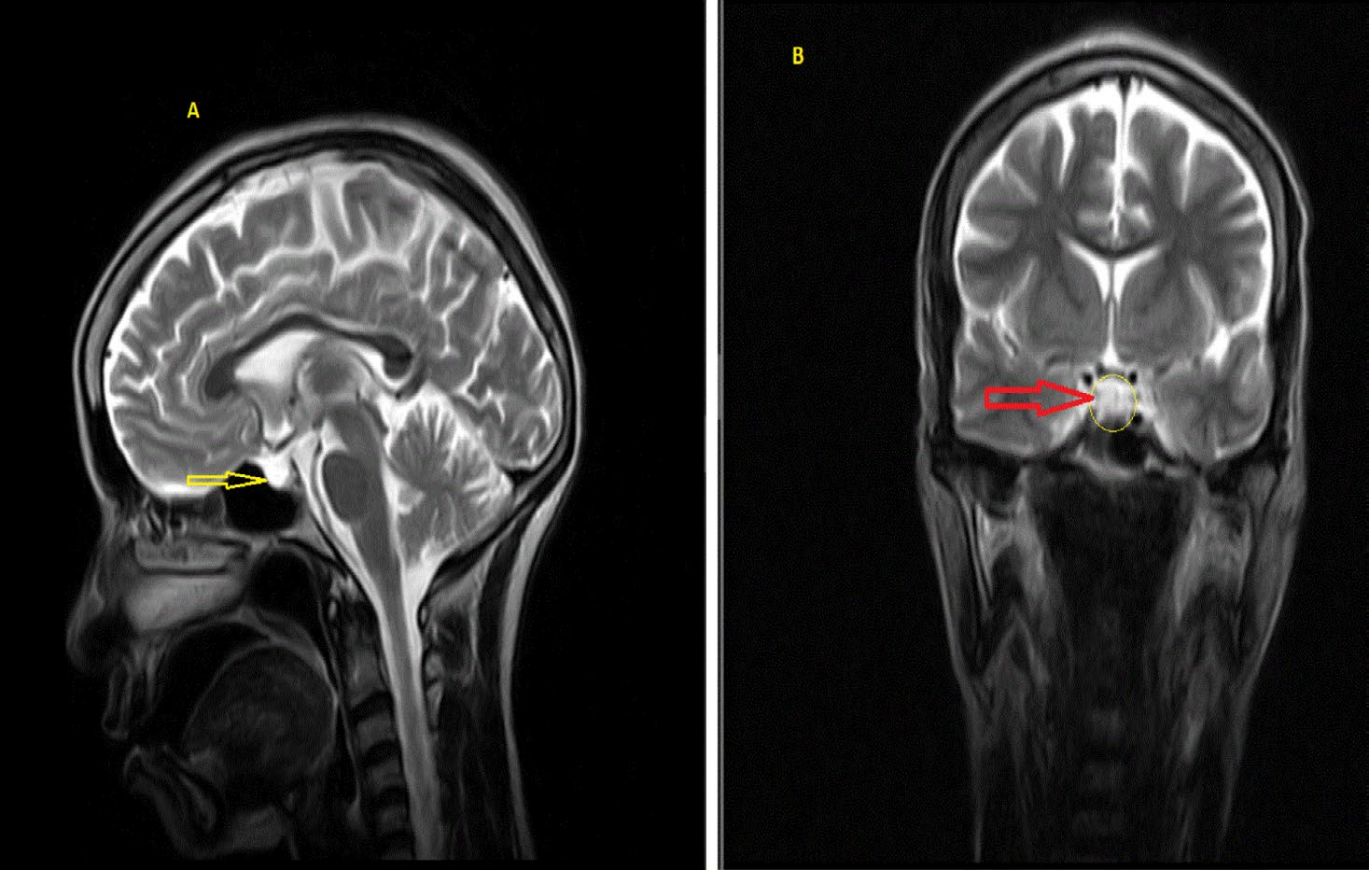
T2‐weighted MRI of the pituitary fossa. (A) Sagittal plane showing the sella turcica region. Note the pituitary tissue is compressed to the sellar floor, with increased cerebrospinal fluid (CSF) signal filling the pituitary fossa (yellow arrow), consistent with a Grade 4 empty‐sella appearance. (B) Coronal plane demonstrating near‐total absence of pituitary tissue, with CSF occupying most of the sella (red arrow and yellow circle). No cystic changes or mass lesions are present.
